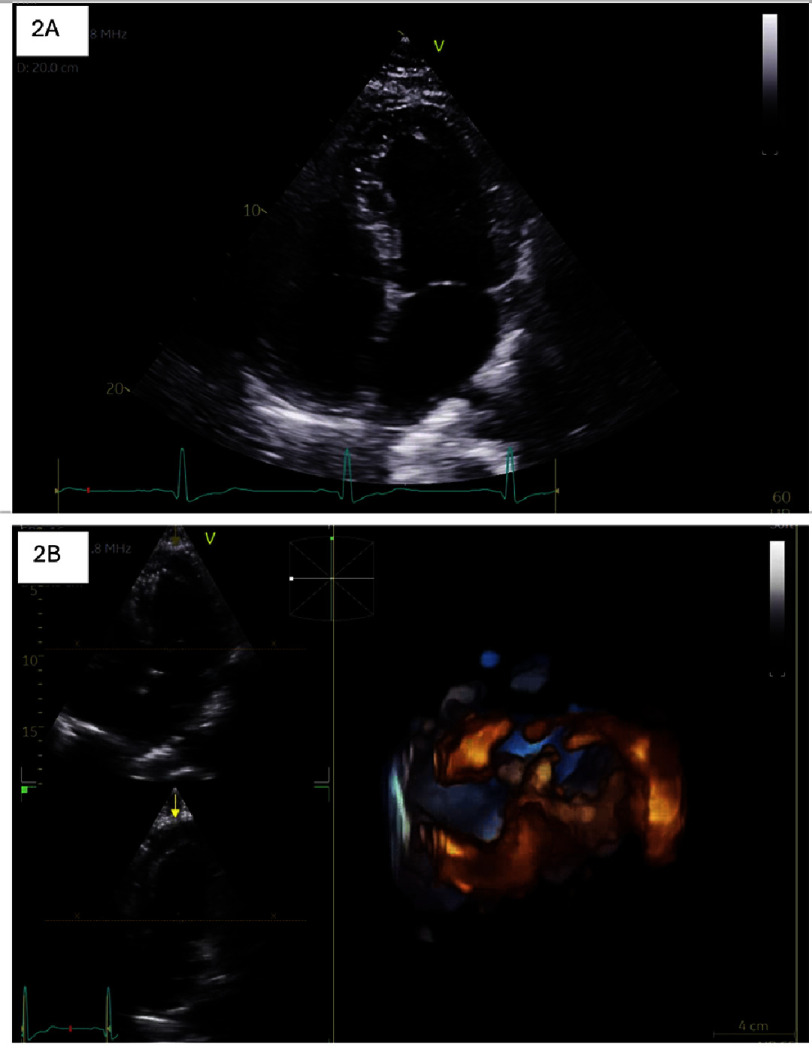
Transthoracic echocardiogram demonstrated normal left and right ventricular size and function. The left atrium was noted to be mildly to moderately dilated.
